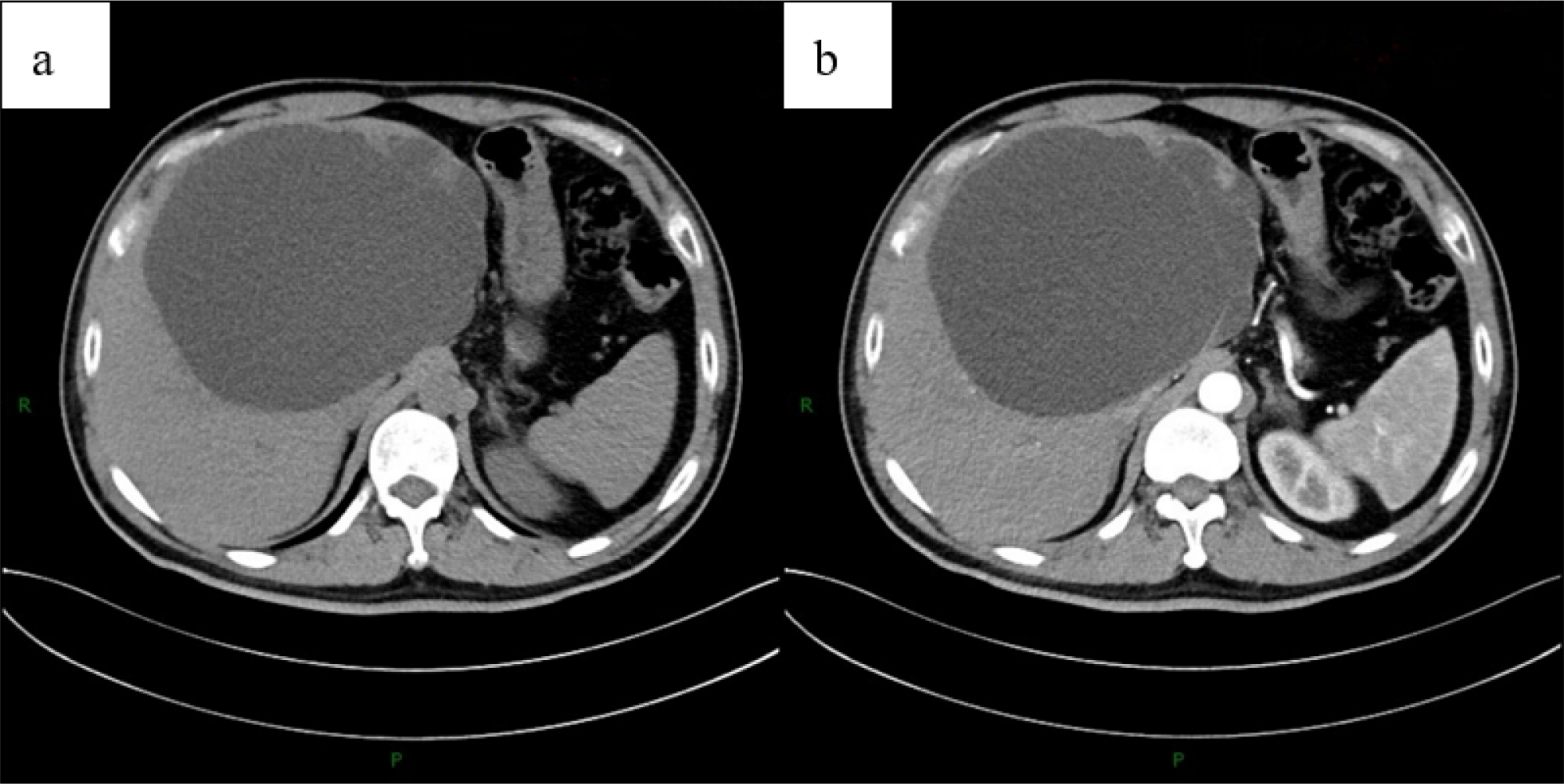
Noncontrast (a) and contrast-enhanced (b) CT of the upper abdomen demonstrate a round, fluid-attenuation lesion in the left hepatic lobe measuring approximately 16.7 x 13.9 cm, without internal enhancement and causing mass effect on adjacent structures, findings compatible with a large hepatic cyst.
